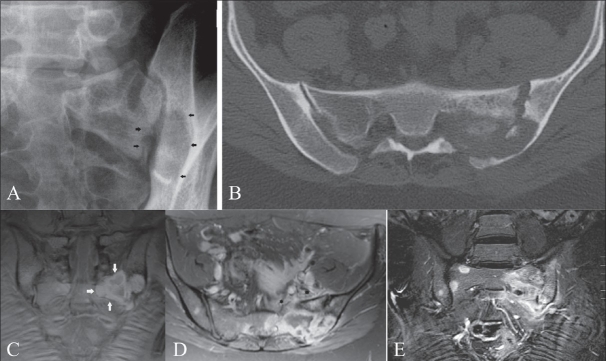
Tuberculous sacroiliac joint infection. Plain film of the left sacroiliac joint (A) shows large erosions with surrounding sclerosis on both sides of the left sacroiliac joint (black arrows). CT scan of the sacroiliac joints (B) confirms marked destruction of the left sacroiliac joint. Coronal fat-suppressed T1W MRI (C) shows a hyperintense peripheral rim around an intraosseous abscess within the left sacrum (arrows). Axial, contrast-enhanced, fat-suppressed T1W MRI (D) shows peripheral enhancement of the large subchondral erosions and adjacent intraosseous abscess. Note the enhancement of the synovium and of the soft tissues anterior to the left sacroiliac joint (asterisks). Coronal, contrast-enhanced, fat-suppressed T1W MRI (E) shows contrast uptake on both sides of the left sacroiliac joint, absence of contrast uptake within the central part of joint and multiple areas of contrast uptake within the sacrum and spine, due to associated disseminated tuberculous osteomyelitis
